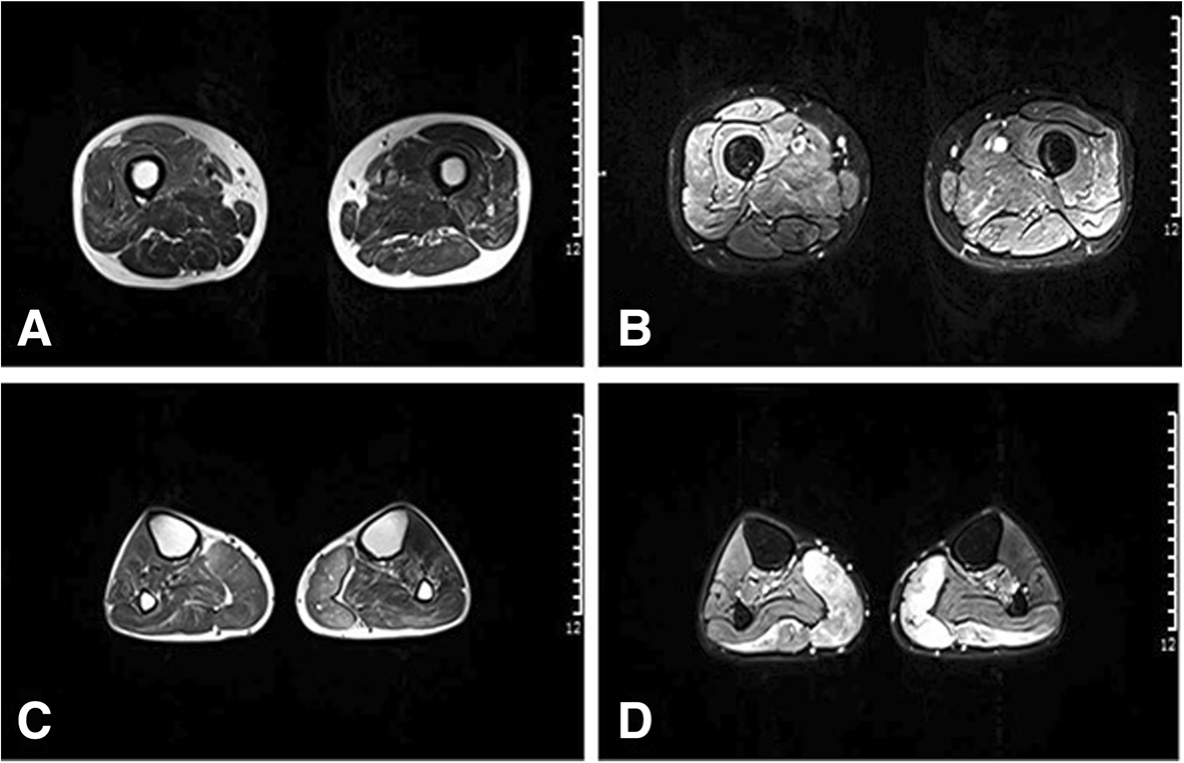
Transverse T2-weighted and TIRM sequence muscle magnetic resonance images. A slightly increased signal was detected in the posterior compartment of the thigh, in the semimembranosus and semitendinosus muscles (a: T2-weighted, b: TIRM). A highly increased signal intensity in TIRM sequences was detected at the level of the lower leg, indicating obvious muscular edema (d). A mild increase in the signal intensity was observed in the T2 sequence of soleus and tibialis anterior muscles, indicating fat replacement (c)
